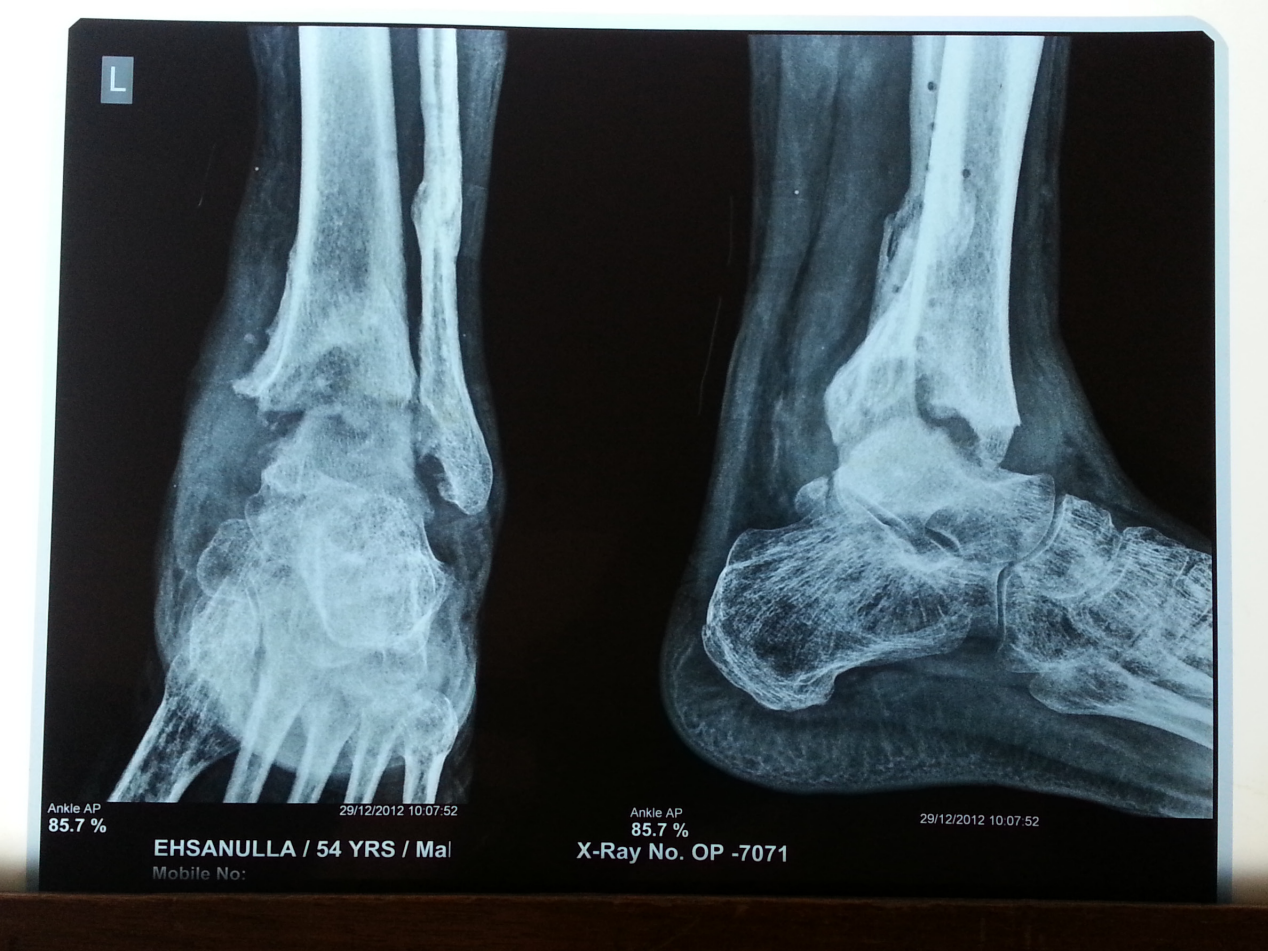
AP and Lat view of Xray showing joint destruction.
